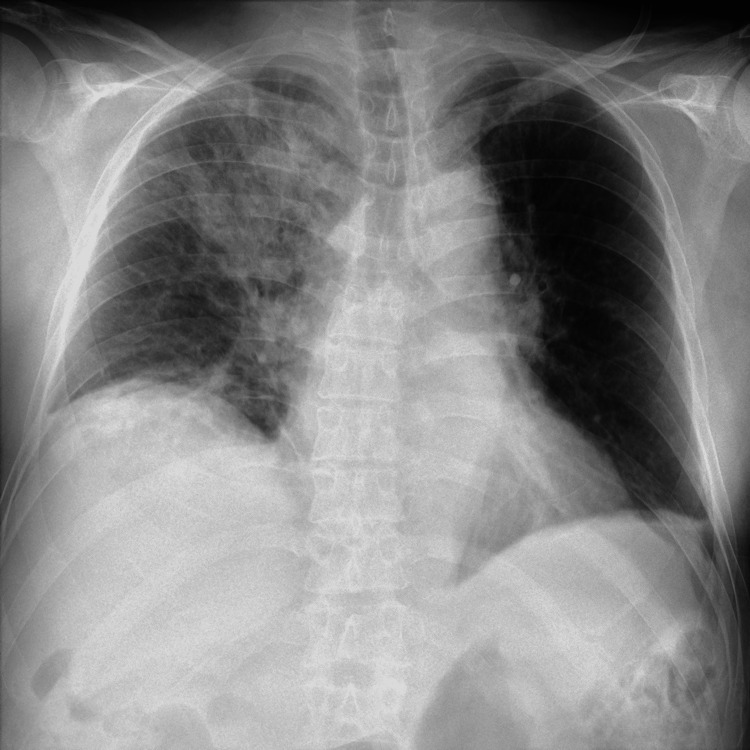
Chest X-ray showing an infiltrate in the right lung involving the perihilar region and upper lobe
